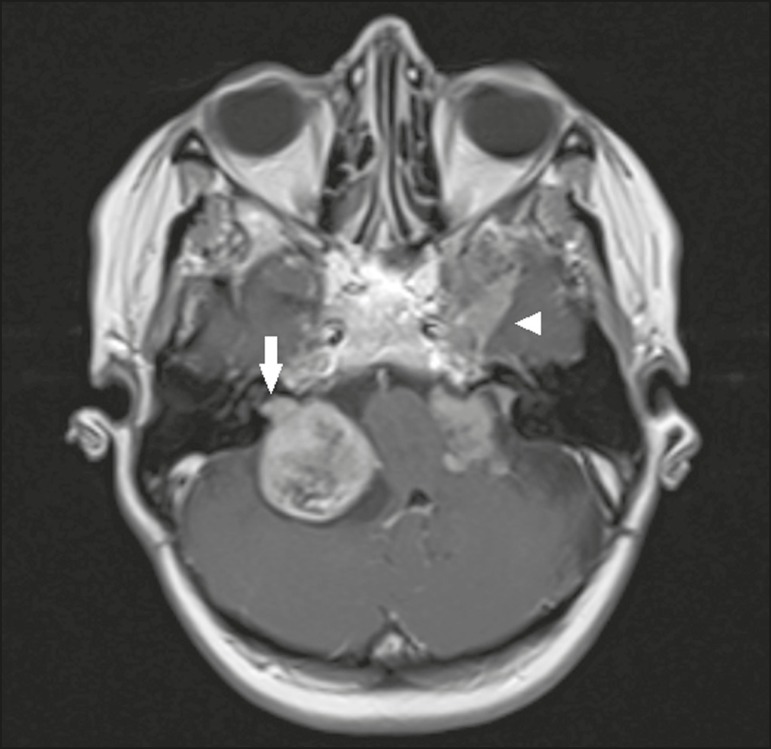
Schwannoma. Contrast-enhanced axial T1-weighted MRI sequence.
